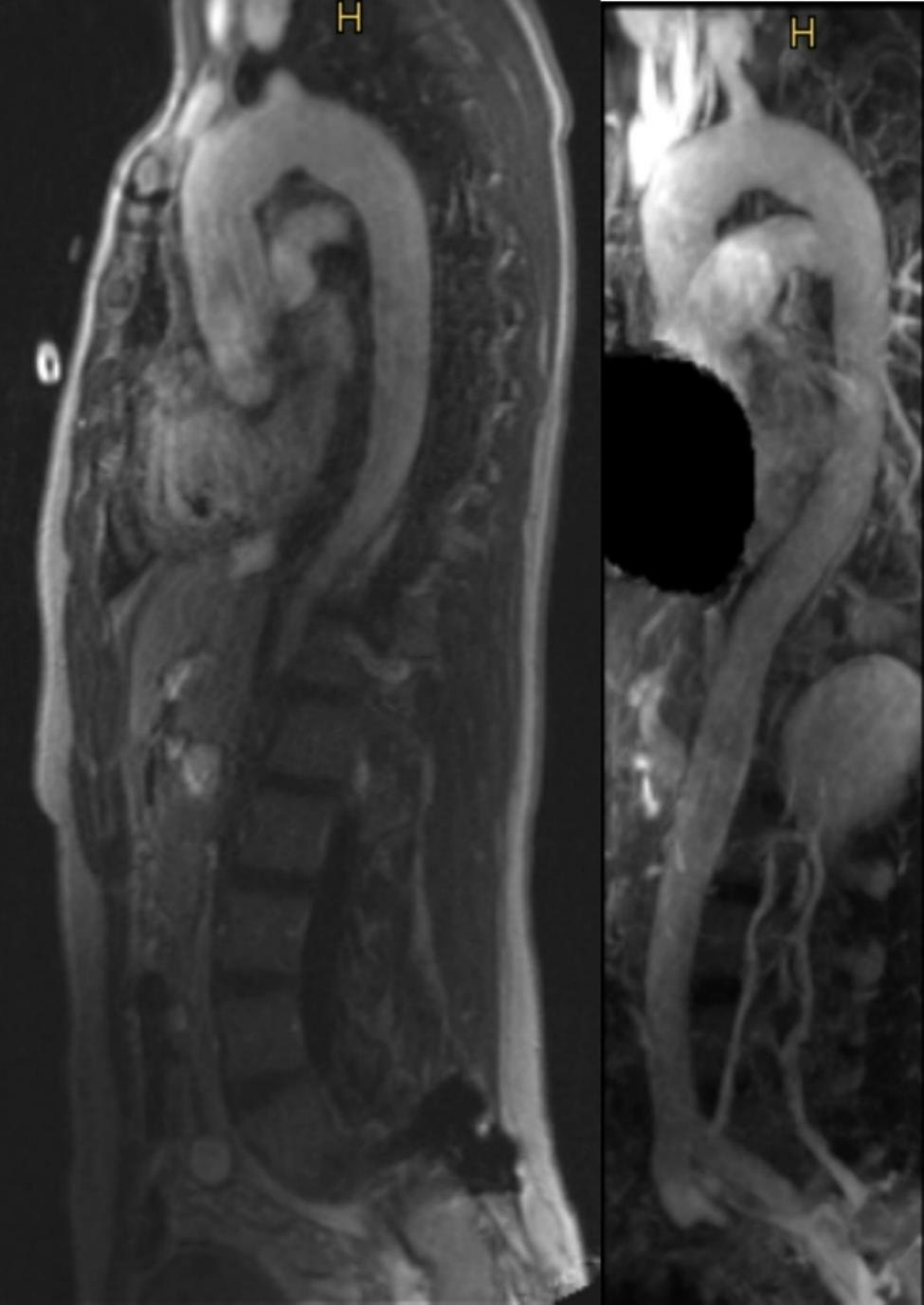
Two years: postoperative MRI control
